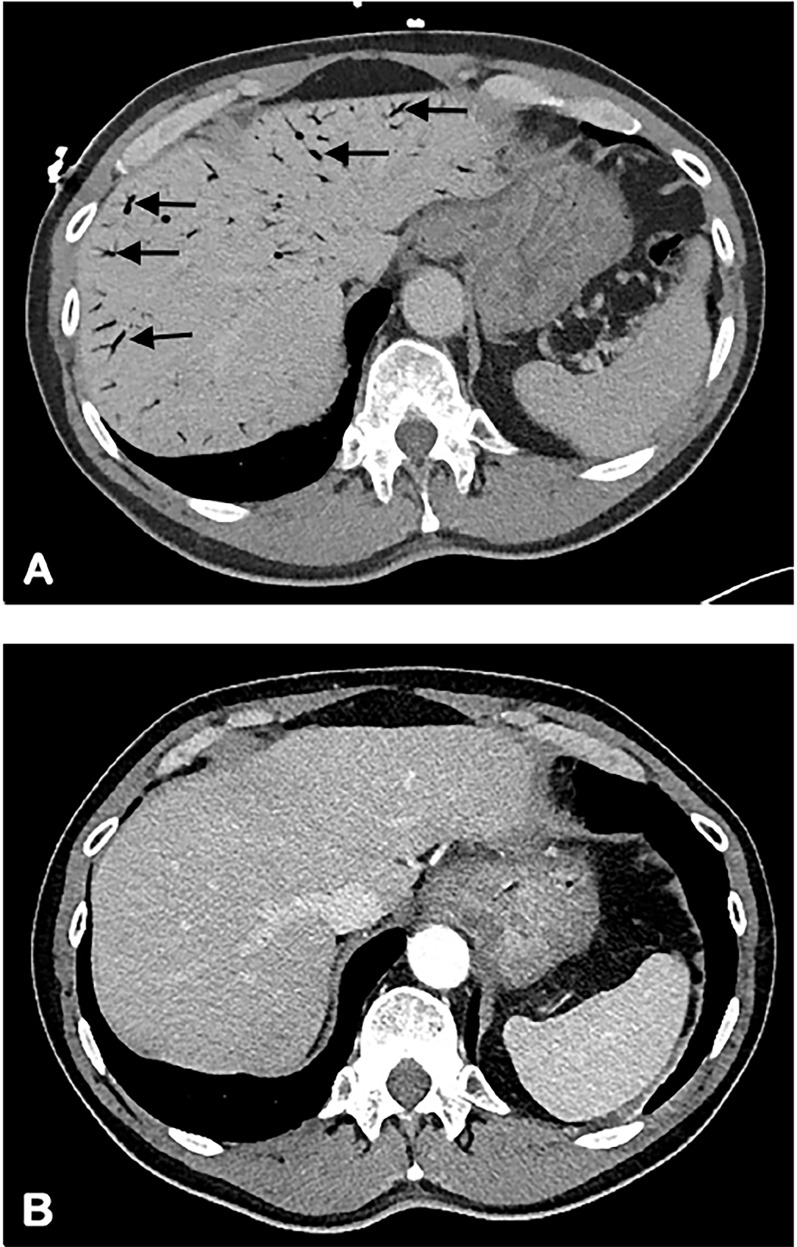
Computed tomography of abdomen showing portal venous gas of the liver before hyperbaric oxygen therapy (arrows) (A) and resolution after therapy (B).
